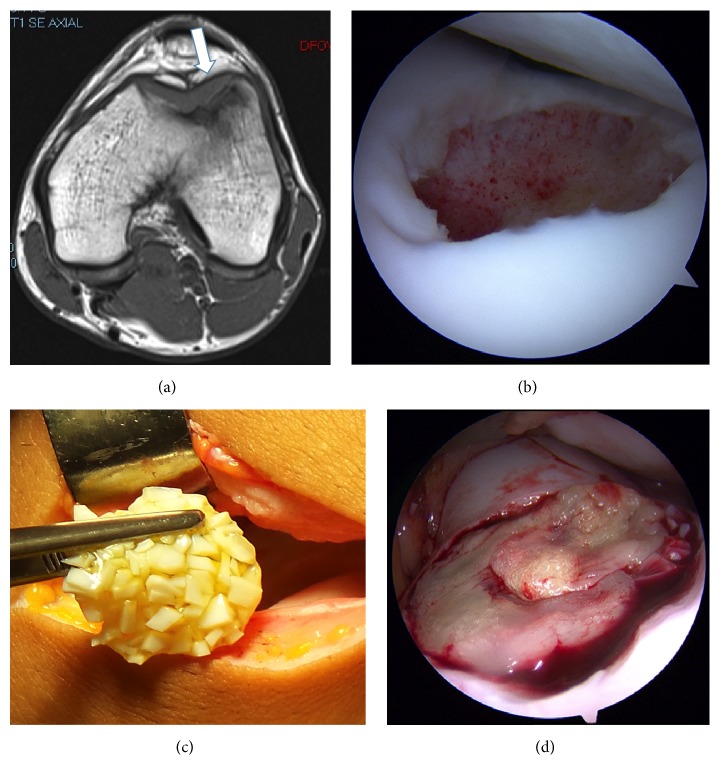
Composition of figures demonstrating the initial injury and surgical treatment. (a) Preoperative axial T1-sequence MRI demonstrating the osteochondral injury (white arrow). (b) Intraoperative picture demonstrating the debrided defect in the lateral femoral condyle. (c) Intraoperative picture demonstrating the autologous matrix before implantation. (d) Arthroscopic view of the filled defect at the end of the procedure.
